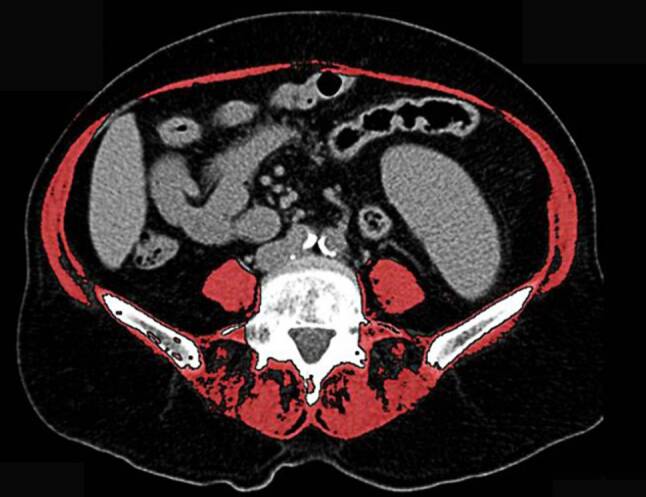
Cross-sectional X-ray image at the L3 level showing measurement of SMI (marked *red*)
